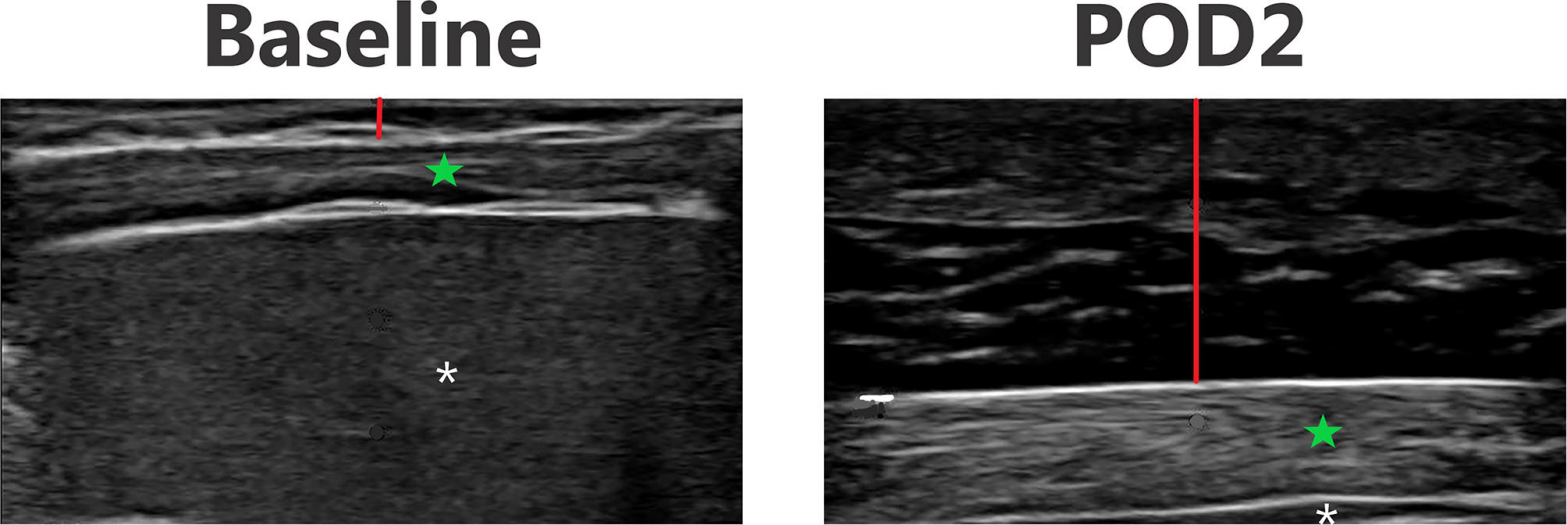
Point-of-care ultrasound images of lateral abdomen at baseline and POD 2 of the same patient. Both images are presented on identical scales (total depth of 2 cm from the skin). Red vertical line demonstrates subcutaneous tissue thickness—there is a marked increase in subcutaneous tissue seen on POD 2. Green star—echogenic muscle changes seen on POD 2. White asterisk—liver.
